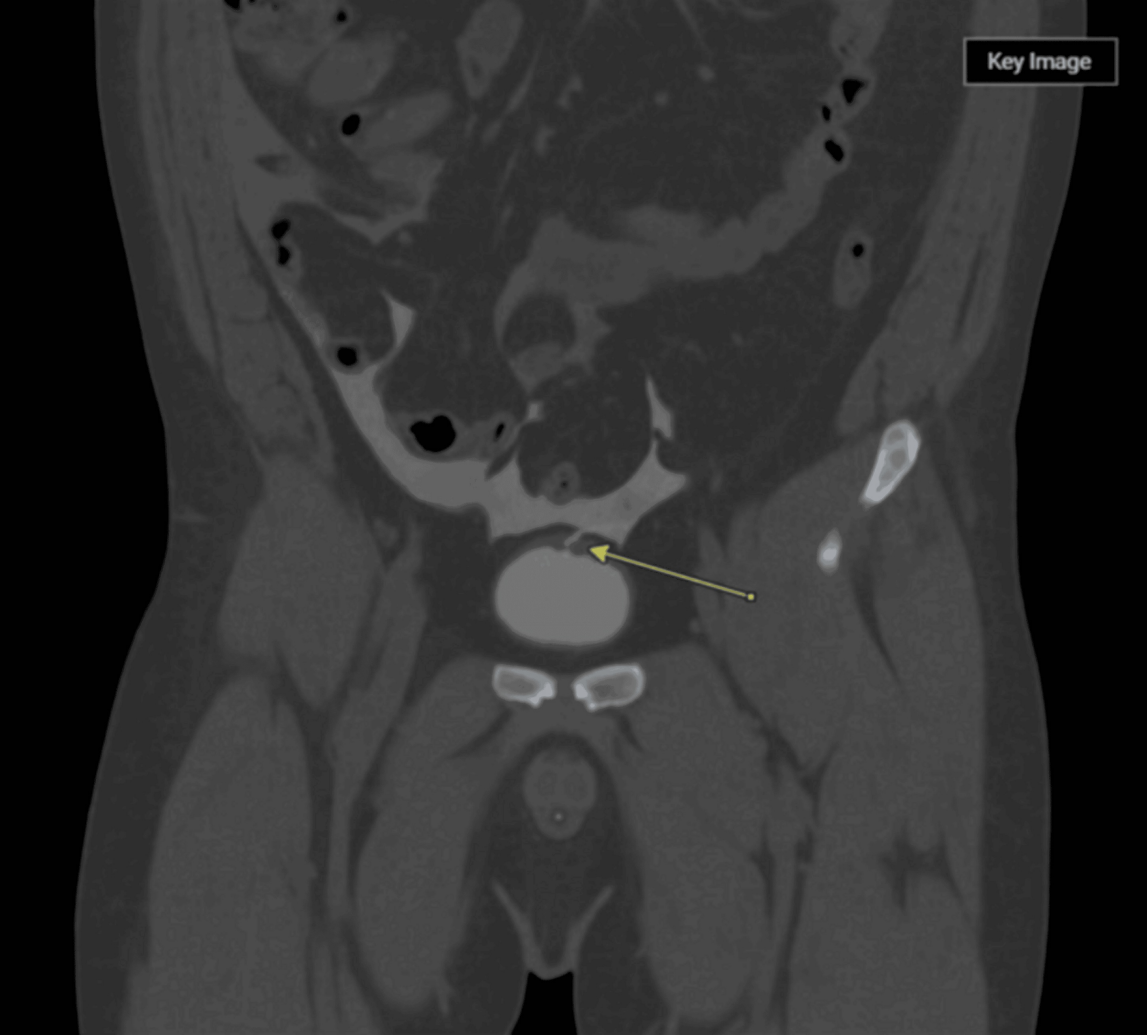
Contrast-enhanced coronal CT scan The yellow arrow is pointing to a defect in the dome of the bladder wall, which appears to be extravasating contrast into the peritoneal cavity, consistent with intraperitoneal BR. BR, bladder rupture
